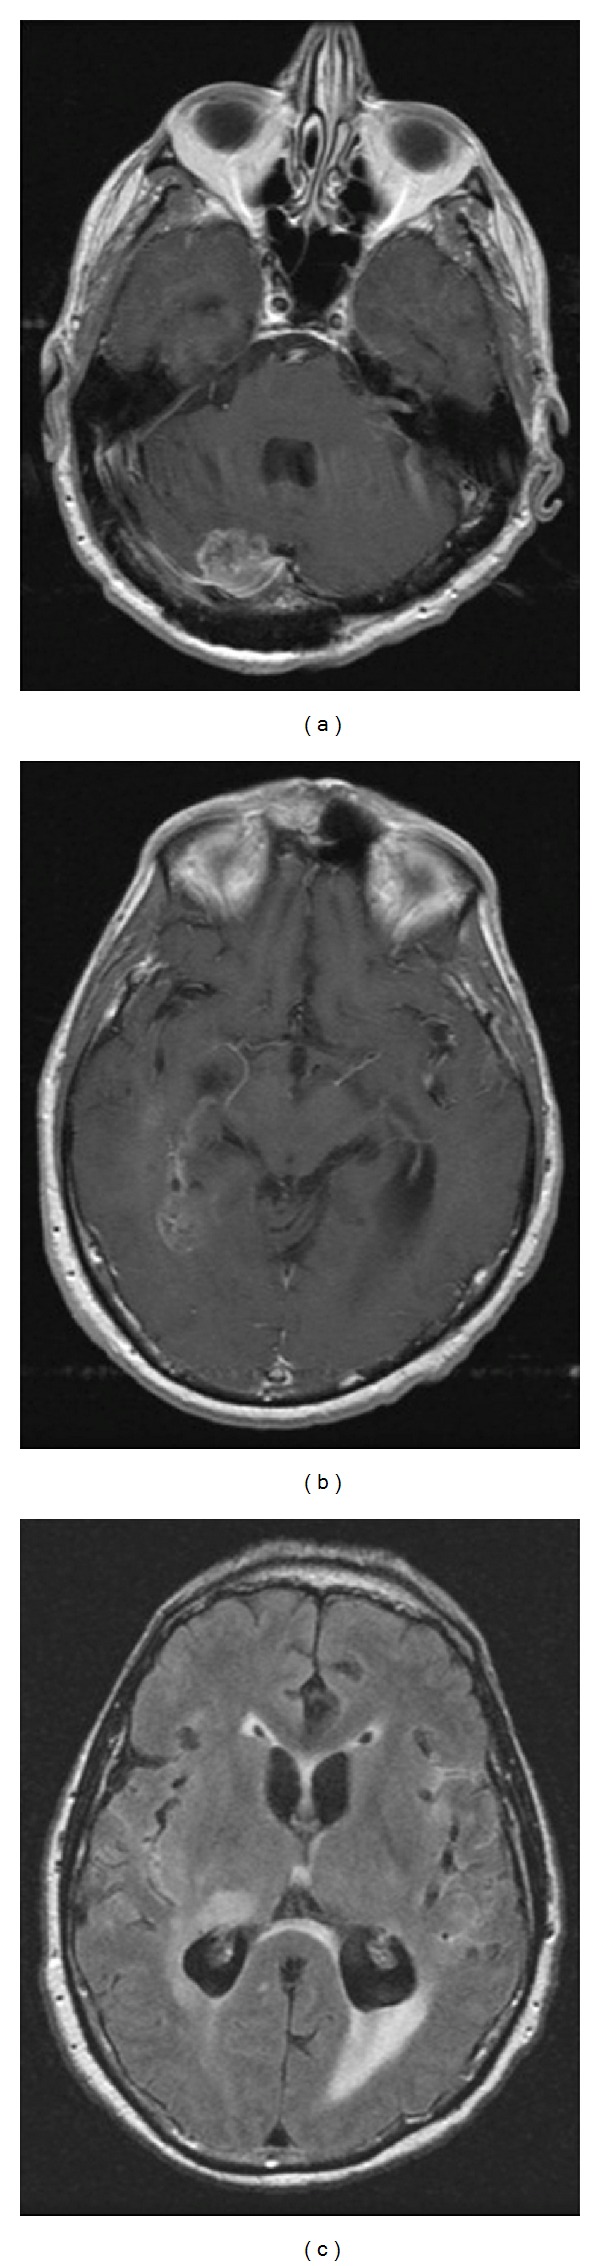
Postoperative MR imaging. (a) Axial T1-weighted image with contrast at the level of the cerebellum displaying subtotal resection of the tumor within the right cerebellar hemisphere. (b) Axial T1-weighted image with contrast at the level of the Sylvian fissures showing leptomeningeal enhancement. (c) Axial FLAIR image above the tentorium revealing diffuse FLAIR signal abnormality within bilateral cerebral hemispheres.
